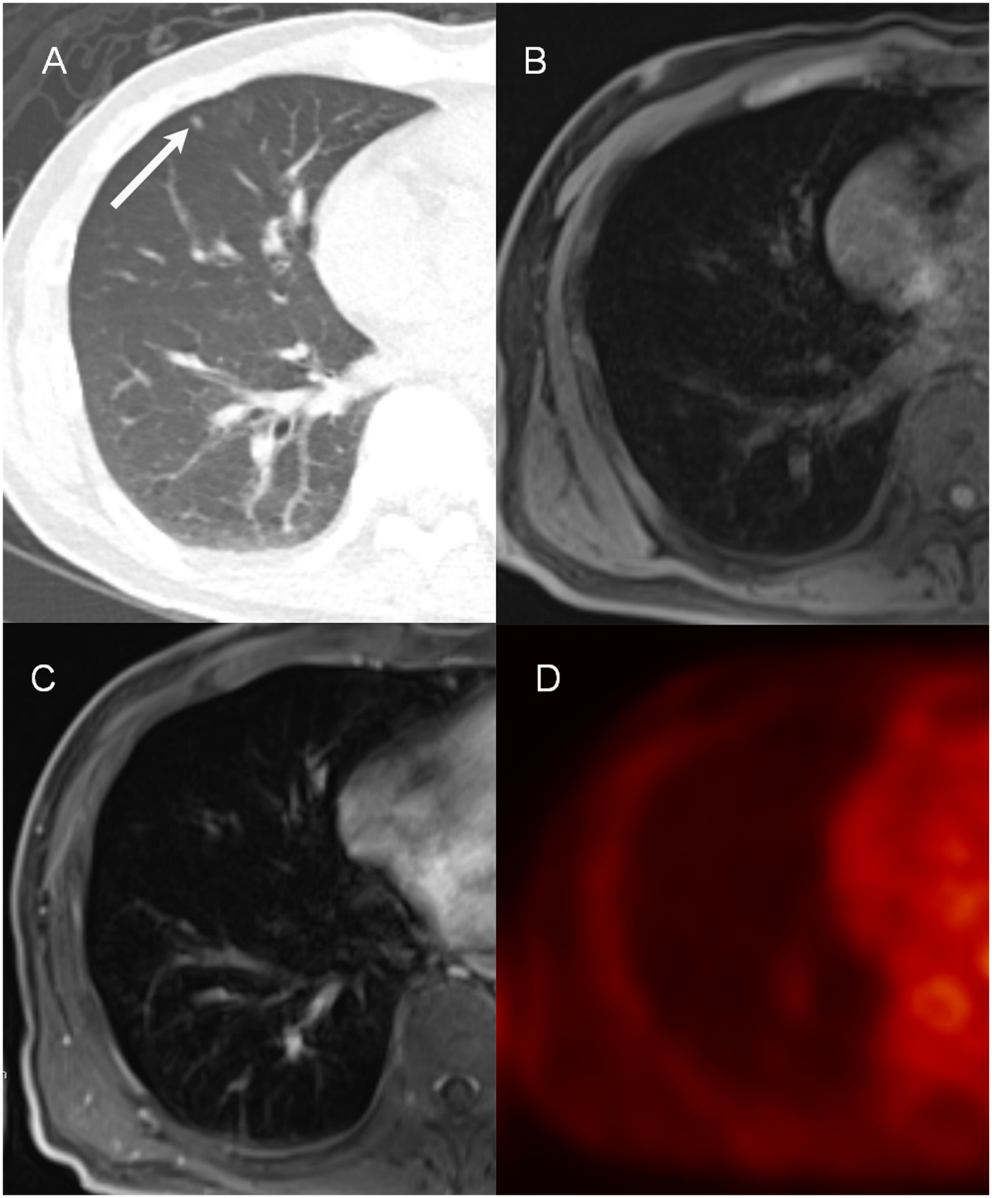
An example of the invisible nodule on PET/MR images (57-year-old man with pancreatic cancer). A tiny nodule (3 mm) is identified (arrow) in the right middle lobe on the CT image (A). This lesion is not visible on pre-contrast VIBE (B) and post-contrast VIBE (C) images. None of the readers were able to identify this nodule during the first and second sessions. This lesion is not visible on PET (D) images due to non-FDG-avidity.
